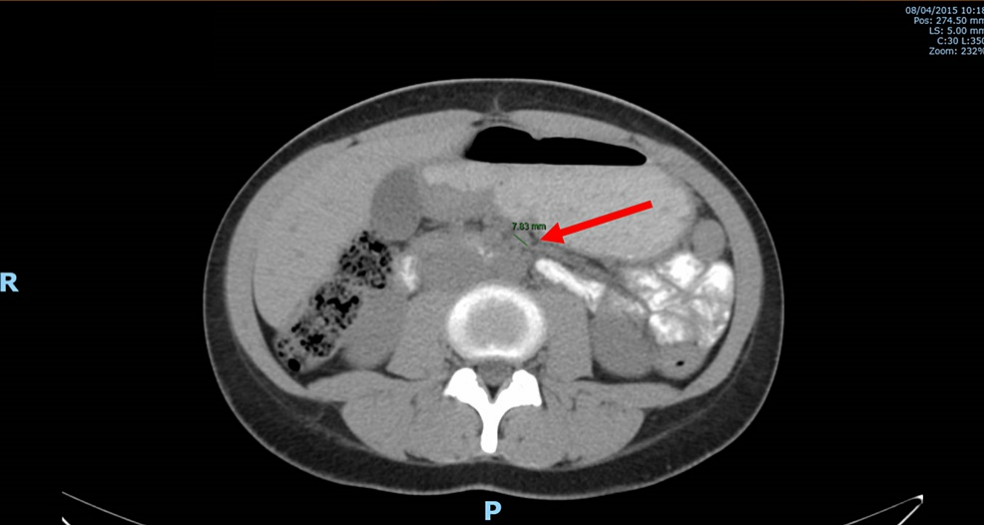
CT scan at diagnosis The initial CT scan showed no signs of metastatic disease, but small nodes were noted in the retrogastric adipose layer, the largest being 7.83 mm in diameter (red arrow) CT: computed tomography
